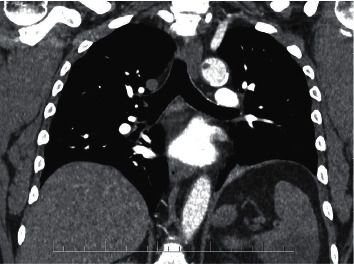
CT angiography showing aortic thrombosis (case no. 1).
